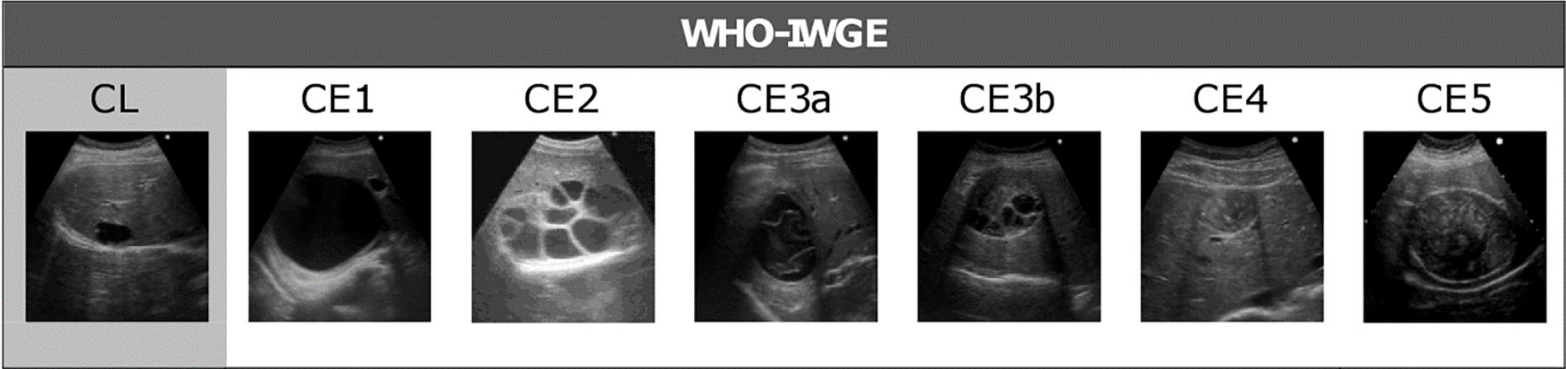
WHO Informal Working Group on Echinococcosis (IWGE) standardized ultrasound classification of echinococcal cysts. CE1 and CE2 (active cysts), CE3a and CE3b (transitional cysts), and CE4 and CE5 (inactive cysts) [32].
